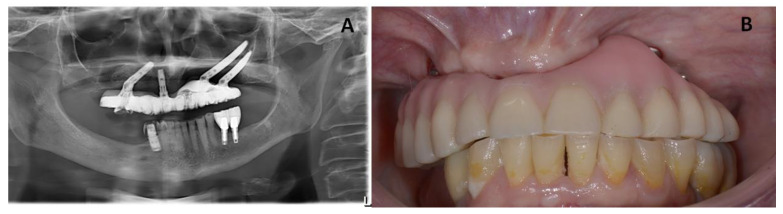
(A) Panoramic radiograph of the patient after 3 years of follow-up. (B) Clinical image from the patient showing occlusion.
